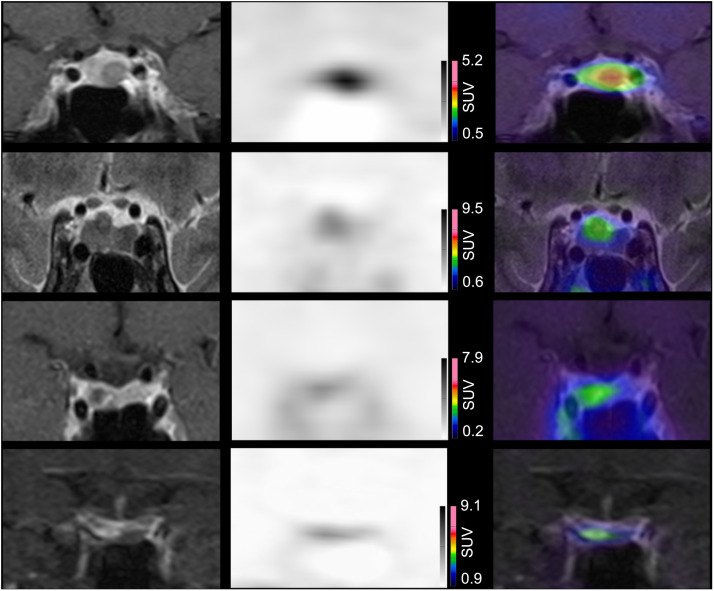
First column shows coronal MR images (first and third rows, T1-weighted time spin echo [TSE] after gadolinium MRI; second row, T2-weighted TSE; fourth row, dynamic T1-weighted TSE). Second column displays corresponding [^11^C]MET PET images. Third column presents superimposed PET and MR images. First row: Patient 4 shows clear left-sided focal uptake, concordant with 8-mm tumor identified on MRI and confirmed by pathology. Second row: Patient 11 shows clear median focal uptake, concordant with 5-mm tumor on MRI and confirmed by pathology. Third row: Patient 29 shows clear right-sided focal uptake, concordant with 6-mm tumor on MRI and pathologic findings. Fourth row: Patient 15 shows right-sided focal uptake not matching 5-mm tumor observed on MRI, and both findings are discordant with pathology.
